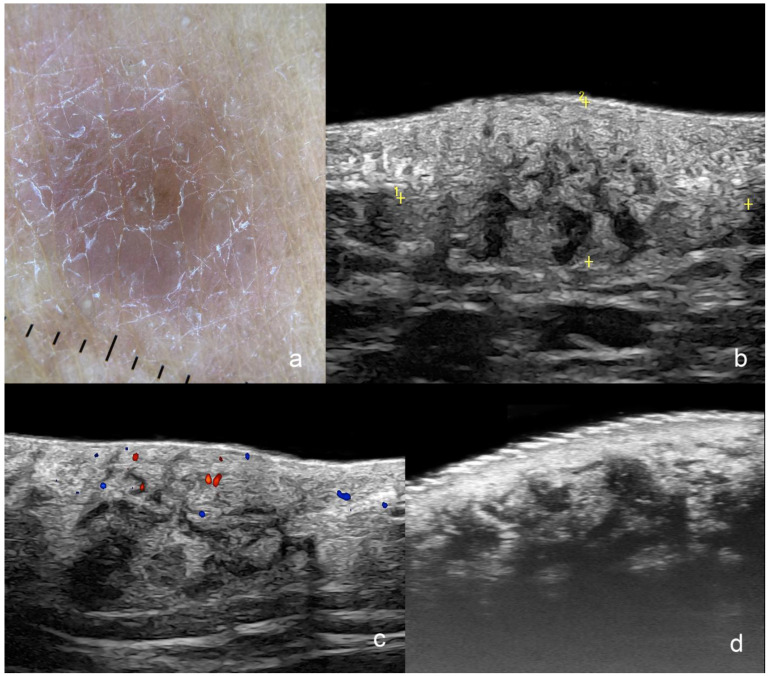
(a–d). Cutaneous Lymphoma. (a). Clinical dermoscopy image at the dorsal region. (b–d). Ultrasound images ((b,c) at 24 MHz; (b), greyscale and (c), color Doppler; longitudinal views; (d), greyscale at 71 MHz; longitudinal view) show a focal dermal and hypodermal infiltrative area with ill-defined borders (between markers) and heterogenous echogenicity that presents some hypoechoic disorganized bands and pseudonodular structures. On color Doppler (c), there is a mild increase in the regional vascularity.
